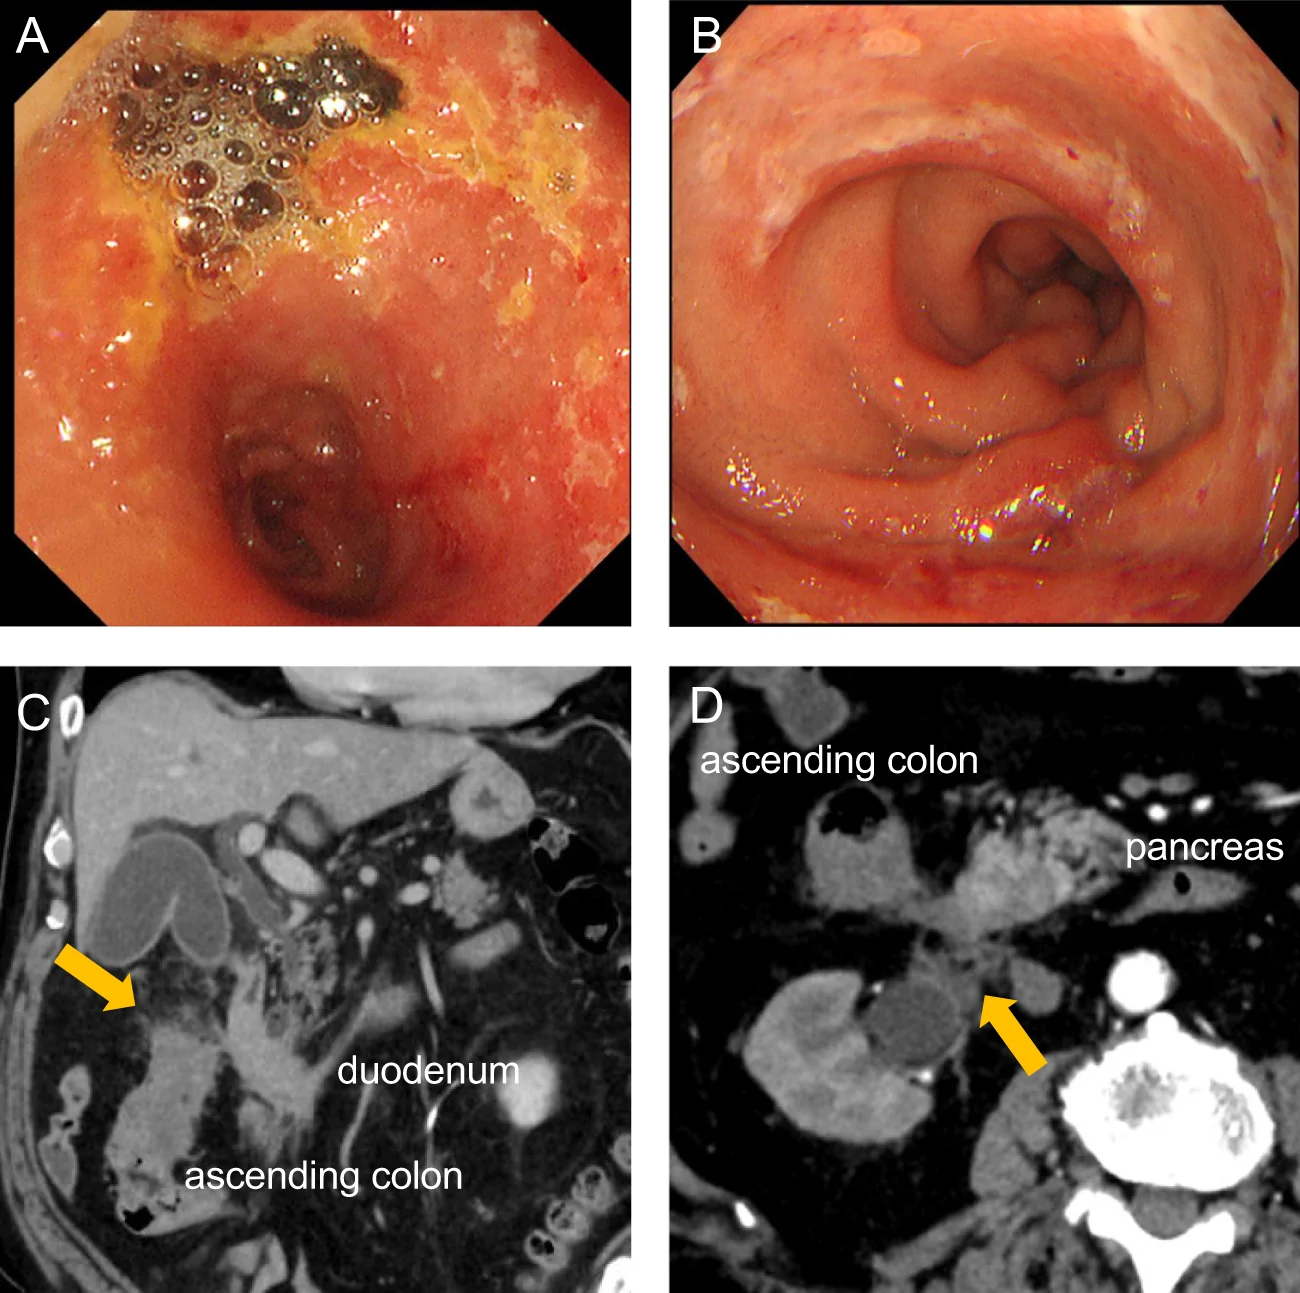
Initial upper gastrointestinal endoscopy and contrast-enhanced CT findings. (a, b) Upper gastrointestinal endoscopy revealed edematous narrowing extending from the duodenal bulb to the descending portion of the duodenum, without any obvious mucosal tumor lesions. (c) Contrast-enhanced computed tomography (CT) demonstrated increased fat attenuation surrounding the duodenum, ascending colon, and the anterior renal fascia (arrow). (d) Dilatation of the right ureter and right renal pelvis was observed, with a transition point located adjacent to the right ureter (arrow).
